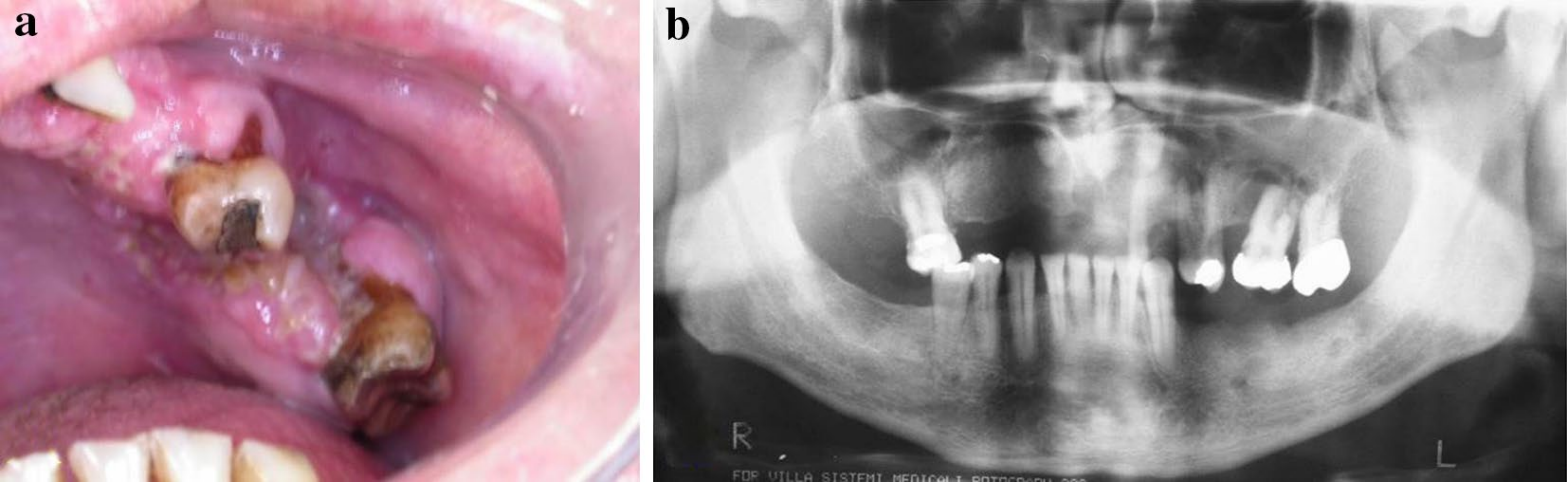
Intraoral (a) and panoramic radiograph (b) showed a lesion around the upper left first and second molars
